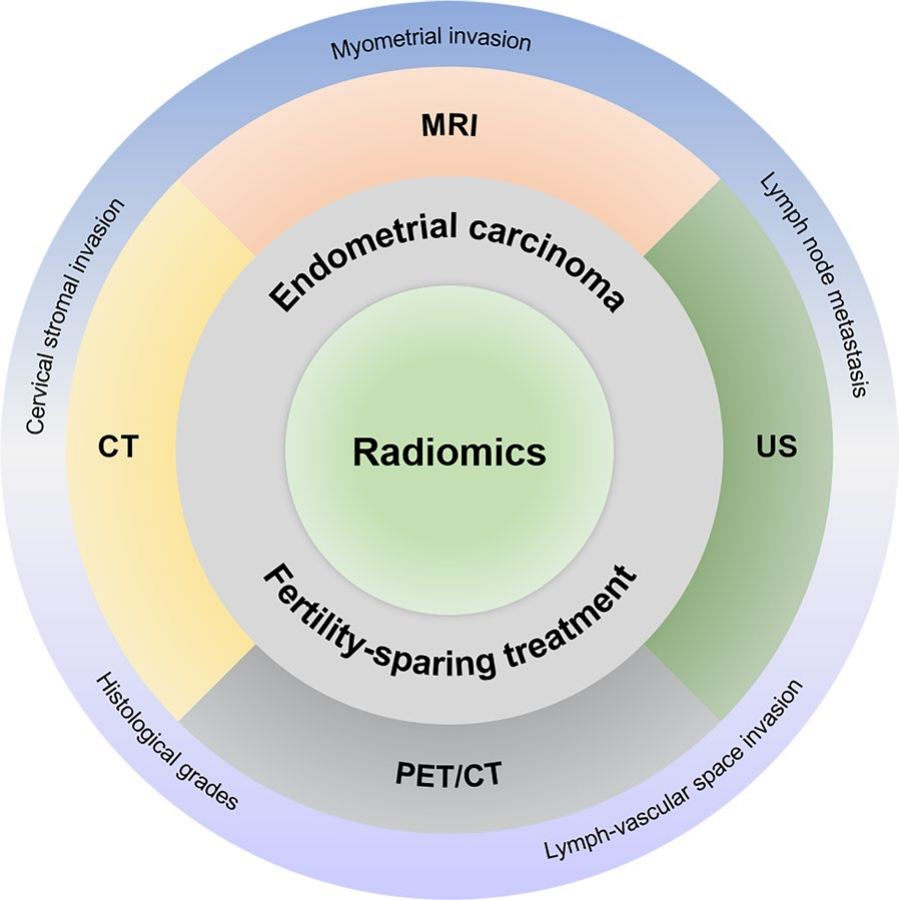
The application of radiomics in fertility-sparing treatment of endometrial carcinoma. MRI = magnetic resonance imaging. US = ultrasound. PET/CT = positron emission tomography/computed tomography. CT = computed tomography
